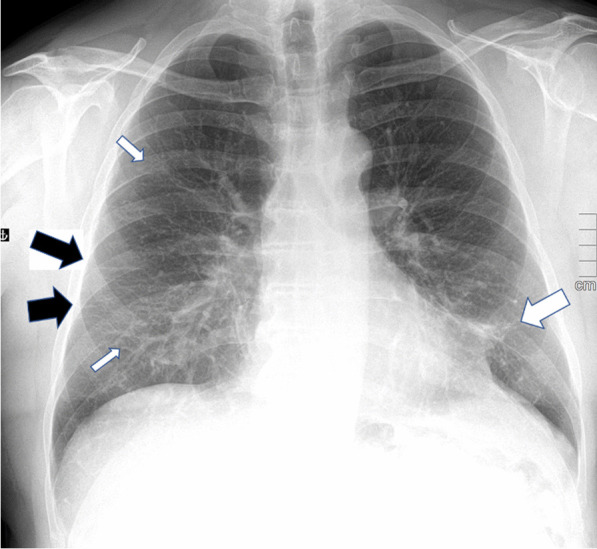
A chest X-ray of an ICU treated patient for COVID-19 showing mainly parenchymal bands (black arrows), faint ground glass opacity (thin white arrows) and consolidation (thick white arrow) 6 months after hospital discharge
